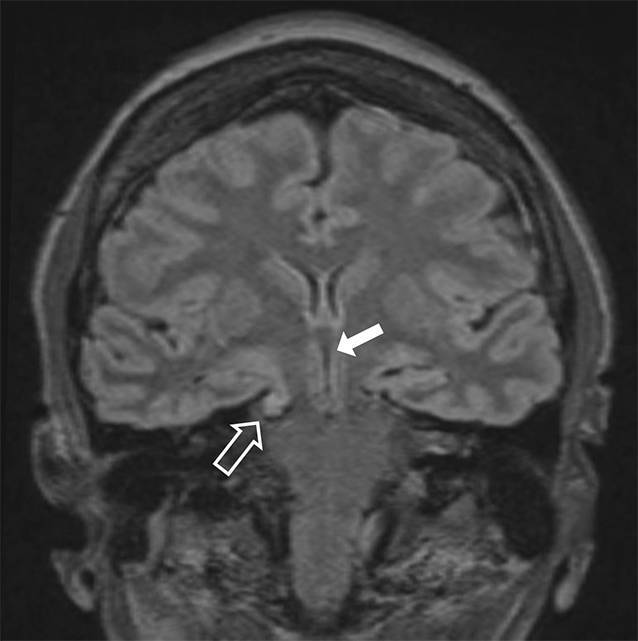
Eine native MRT der spinalen Achse konnte kein Liquorleck detektieren. Bei kaudal verlagertem Hirnstamm und aufgebrauchten infratentoriellen Liquorräumen wurde bei erhöhtem Risiko einer Herniation auf eine invasive Diagnostik mittels CT-Myelographie oder MR-Myelographie ebenso wie auf eine Liquorpunktion zur Druckmessung verzichtet.
